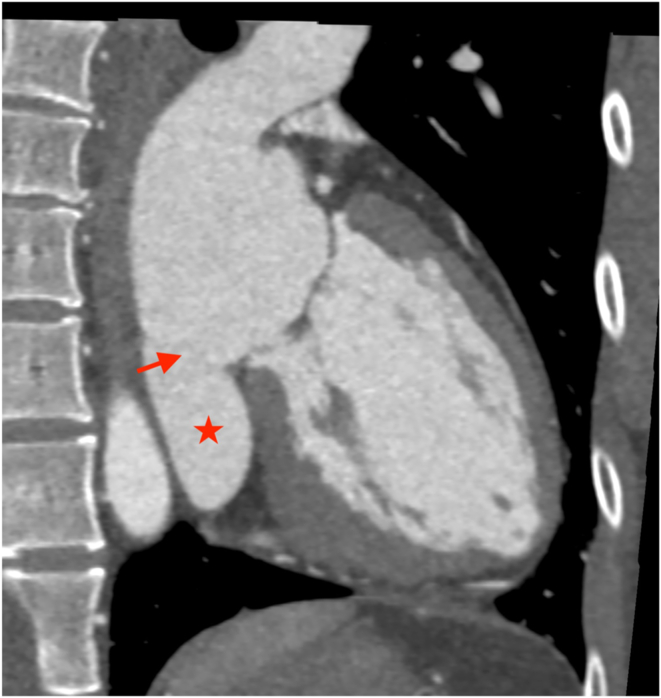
Cardiac computed tomography, oblique sagittal plane, cardiac 2-chamber view, demonstrates the absence of inferior LA “floor” *(arrow)* with LA-CS communication and severely dilated CS *(star)*.
